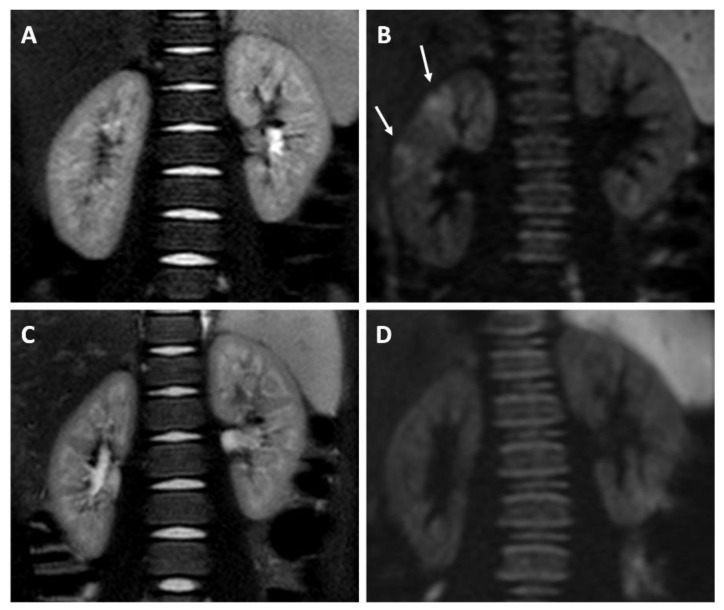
Patient with fUTI. (A) T2-MR sequence shows no signs of acute parenchymal involvement and renal scarring at the moment of the acute episode; (B) signs of acute parenchymal involvement detected by DWI (*b*-value diffusion = 1000); (C) T2-MR sequence shows no sequelae 6 months after the end of treatment; (D) resolution of acute lesions 6 months after the end of treatment, as detected by DW-MRI (*b*-value diffusion = 1000).
